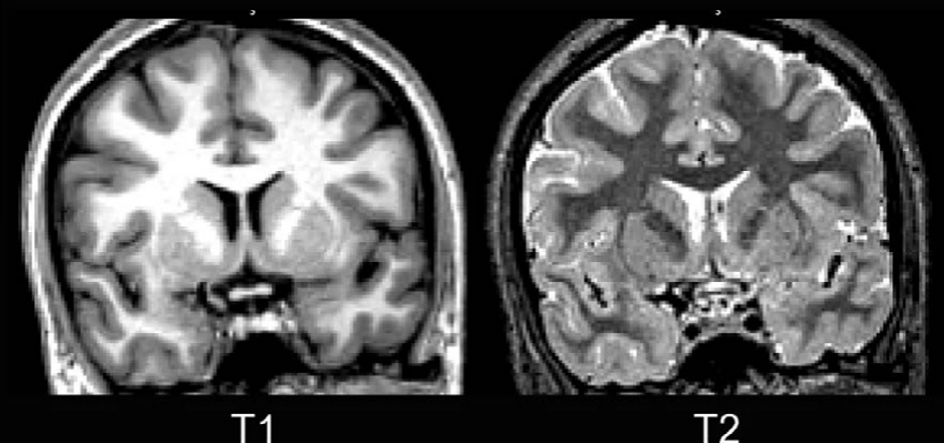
T1- and T2-weighted brain scans. Here, two coronal images from the same young female (age 20 years) are shown in the coronal view. As can be seen, they show inverse intensities. In the T1-weighted image (left panel), compartments with greater water contents appear darker, so that cerebrospinal fluid (CSF) has the darkest intensity, gray matter (for instance in the cortical ribbon, basal ganglia and hippocampi) intermediate intensities, while white matter shows brighter intensities. Such images are often used for reconstruction and quantitative analyses of subcortical and cortical structures. The T2-weighted image (right panel) show inverse intensities with white matter appearing darker, and may be useful for visualizing features such as white matter lesions. When using both types of scans in combination, and T1/T2 ratios, contrast can be improved, and this can be used for myelin mapping (see also Fig. 7).
